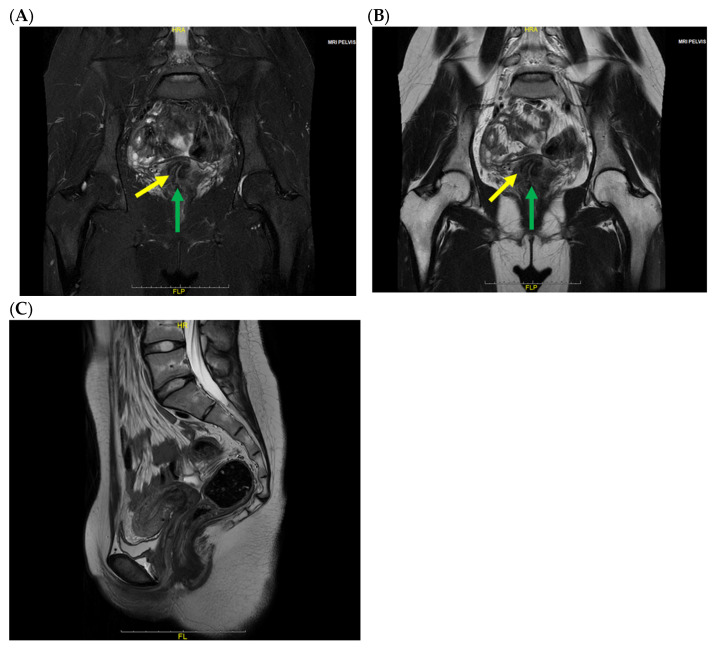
Coronal fat-saturated T2-weighted (A), Coronal T2-weighted (B) and Sagittal T1 weighted (C) MRI one year after surgery show no cavity which connected to the left side of uterine cervix and no obstructed hemivagina and vaginal septum. (Yellow arrow, right uterine cervix; green arrow, left uterine cervix).
